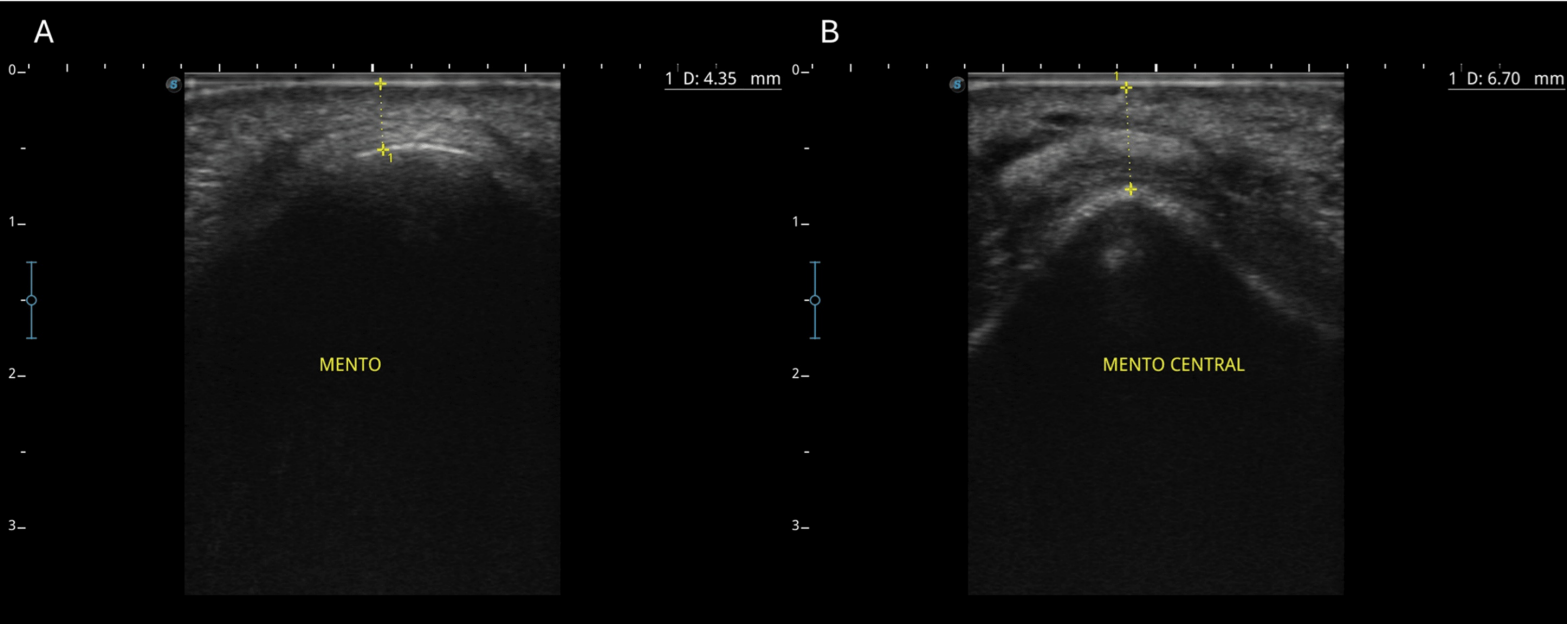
Ultrasonographic evaluation of soft tissue thickness in the menton region before and after treatment. (A) Baseline image (day 0) showing the soft tissue thickness of 4.35 mm. (B) Post-treatment evaluation at 90 days (day 90) showing the thickness of 6.70 mm, confirming a net increase of 2.35 mm and stable filler integration
